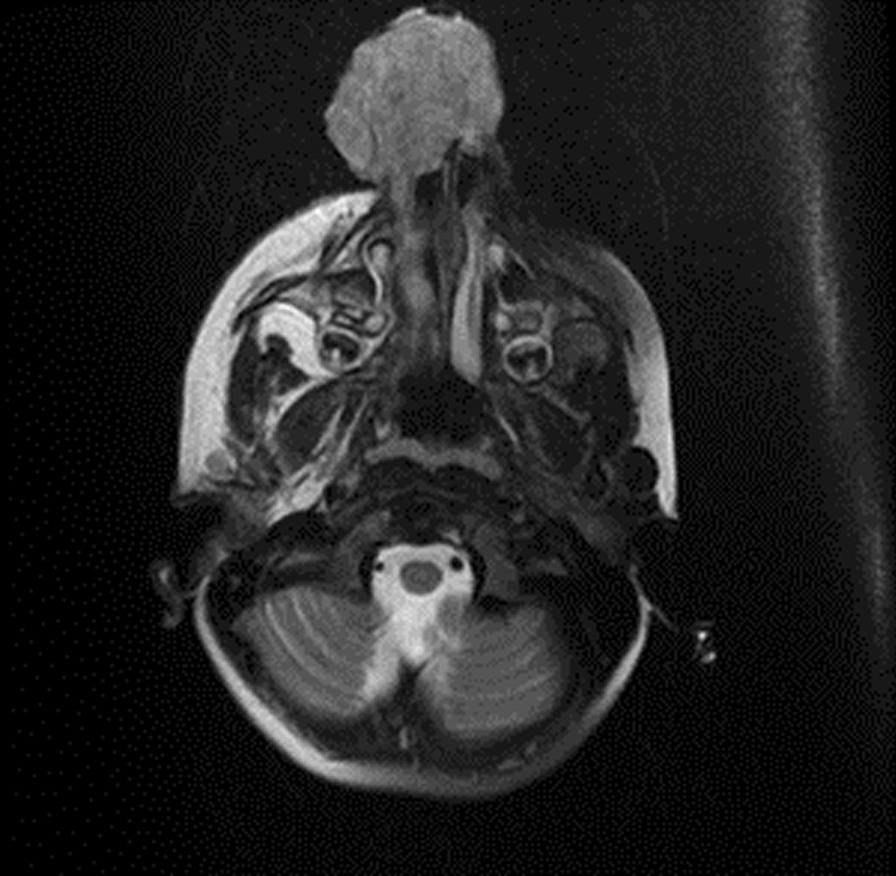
Brain magnetic resonance imaging T2 fat-saturated; a skin-derived lobulated mass with a vertical length of approximately 7.4 cm and transverse dimensions of 4.6 × 4.3 cm at the level of the right nasal aperture at its widest point is observed. Invasion of the mass into the nasal passage or ethmoid cells was not detected
